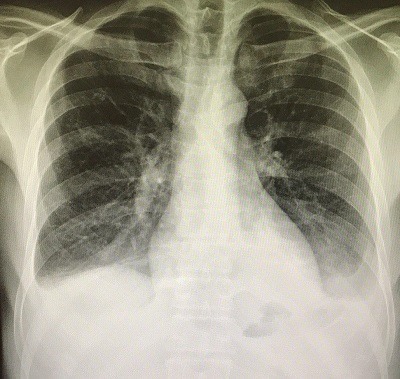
Third X-ray was 6 days after the second operation, omental buttressing, that showing no air or fluid in abdominal cavity
